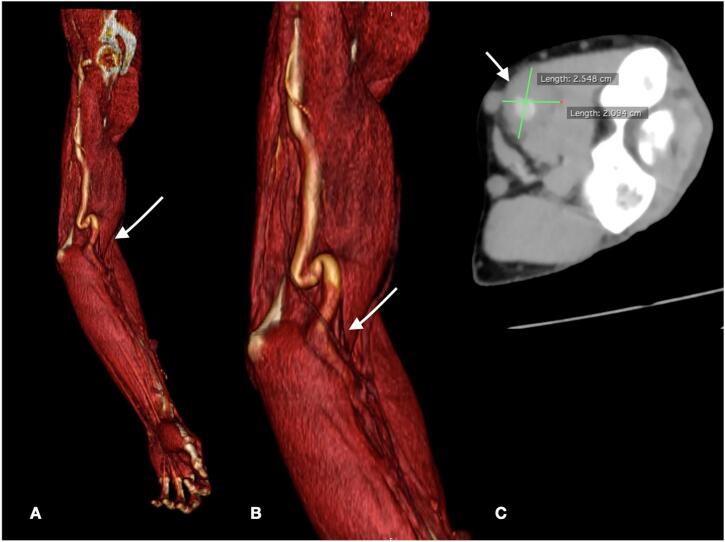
CT Angiography 3-dimensional volume rendering showing left arm (A) with tortuosity and extension of brachial aneurysm (B). CT Angiography with maximum diameter of aneurysm.
